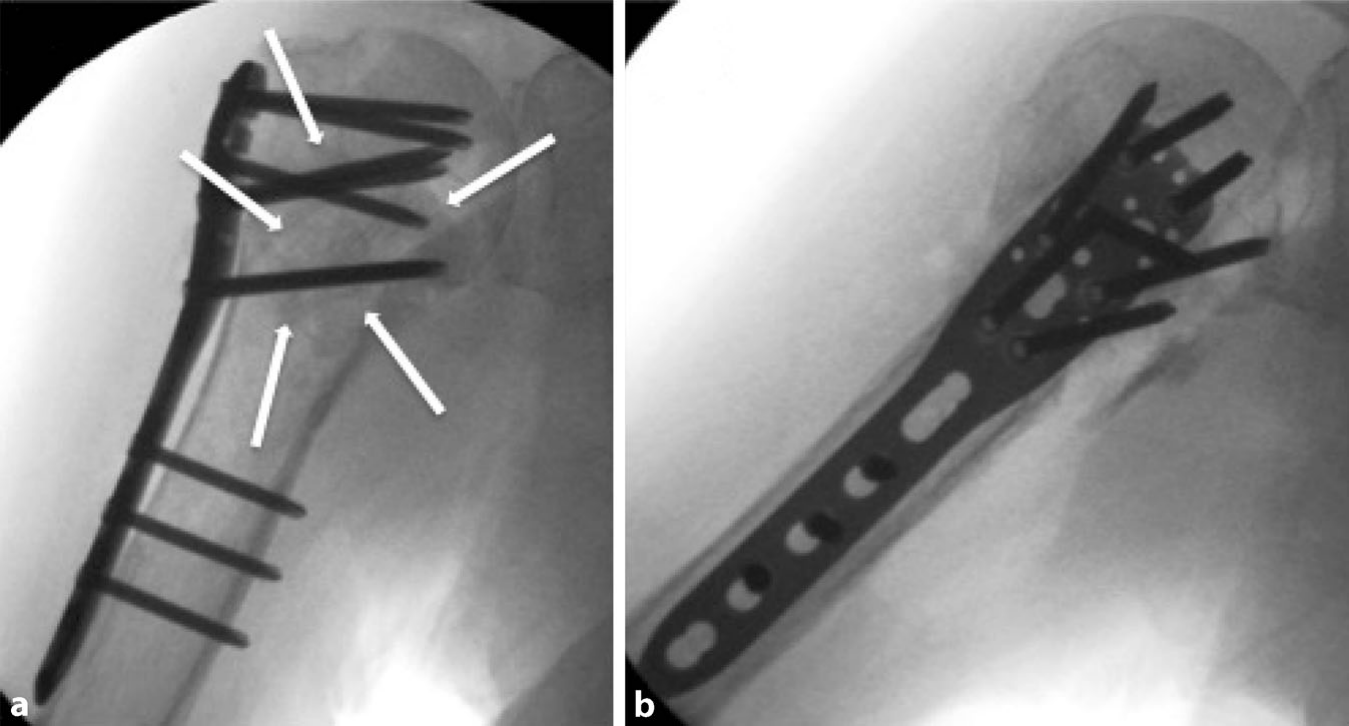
Radiological documentation. Anteroposterior (a) and axial (b) fluoroscopic views are obtained to document the proper position of the allograft and the fracture reduction. After copious lavage and hemostasis, the skin is closed in layers (subcutaneously and the skin itself, additional closure of deep structures like muscles or fascias is not recommended). Adhesive dressing, shoulder sling until interscalene block is dissolved, and for comfort afterwards (see Fig. 15 for details). Anteroposterior (a) and axial (b) fluoroscopic radiographs, intra- (post-)operatively. *White arrows* indicate allograft
